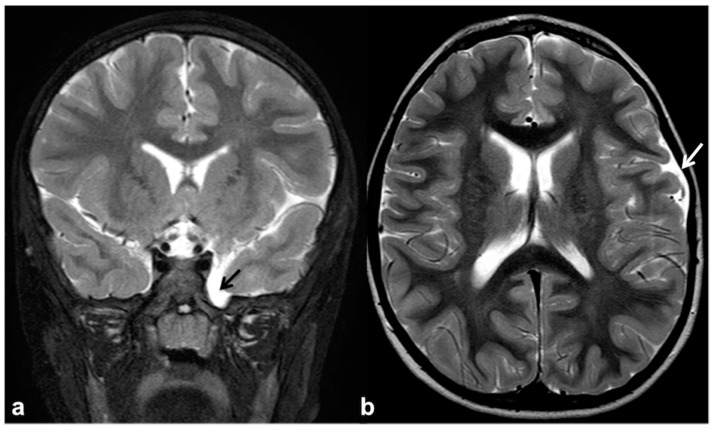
Coronal TSE T2w MRI (a) of a 14 year-old girl with NF1, showing left lateral sphenoid meningocele with no associated brain herniation (black arrow). Axial TSE T2w (b) of the same patient also showed a smaller dural ectasia of the cranial vault, close to the left temporo-parietal suture (white arrow).
